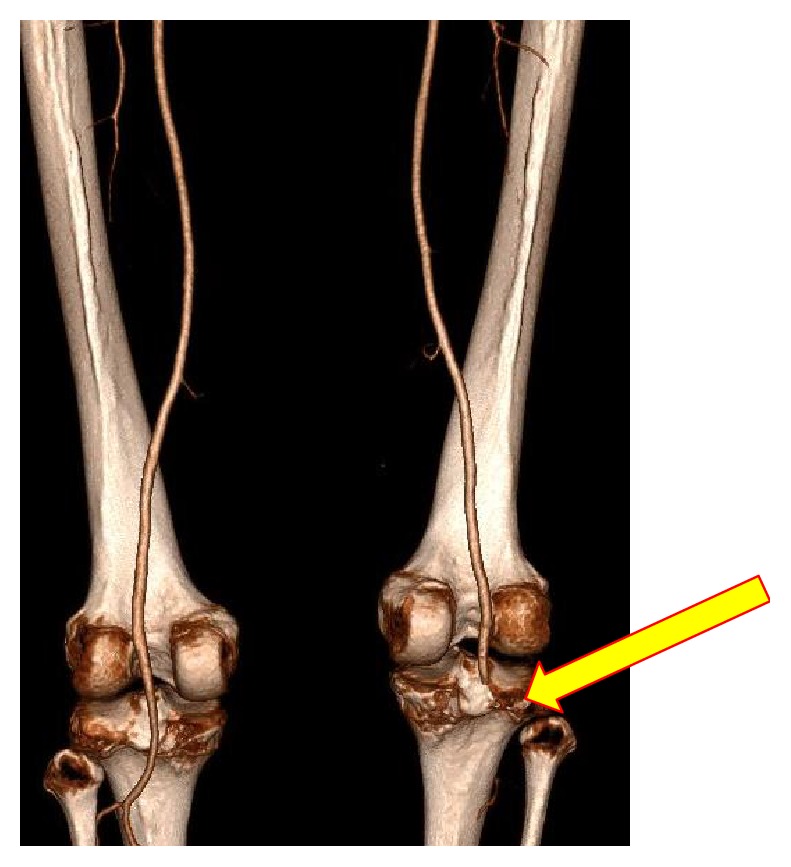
CT peripheral angiogram (25th of May 2016 @ 15:37), 3D reconstruction, posterior view displaying normal vasculature on left, and occlusion to right popliteal artery.
